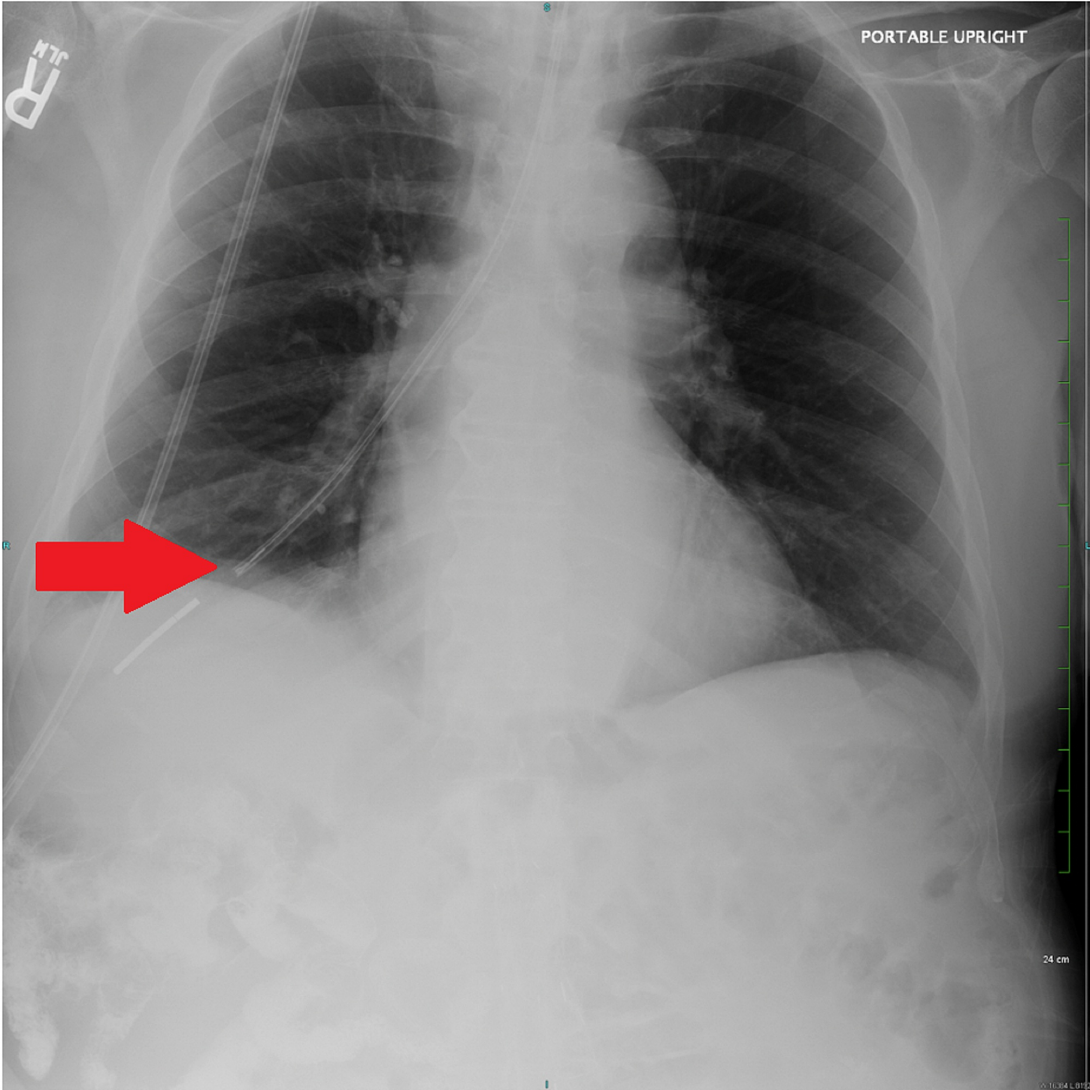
Chest X-ray demonstrating the Dobhoff tube penetrating the right lung base via the right mainstem bronchus (red arrow).
